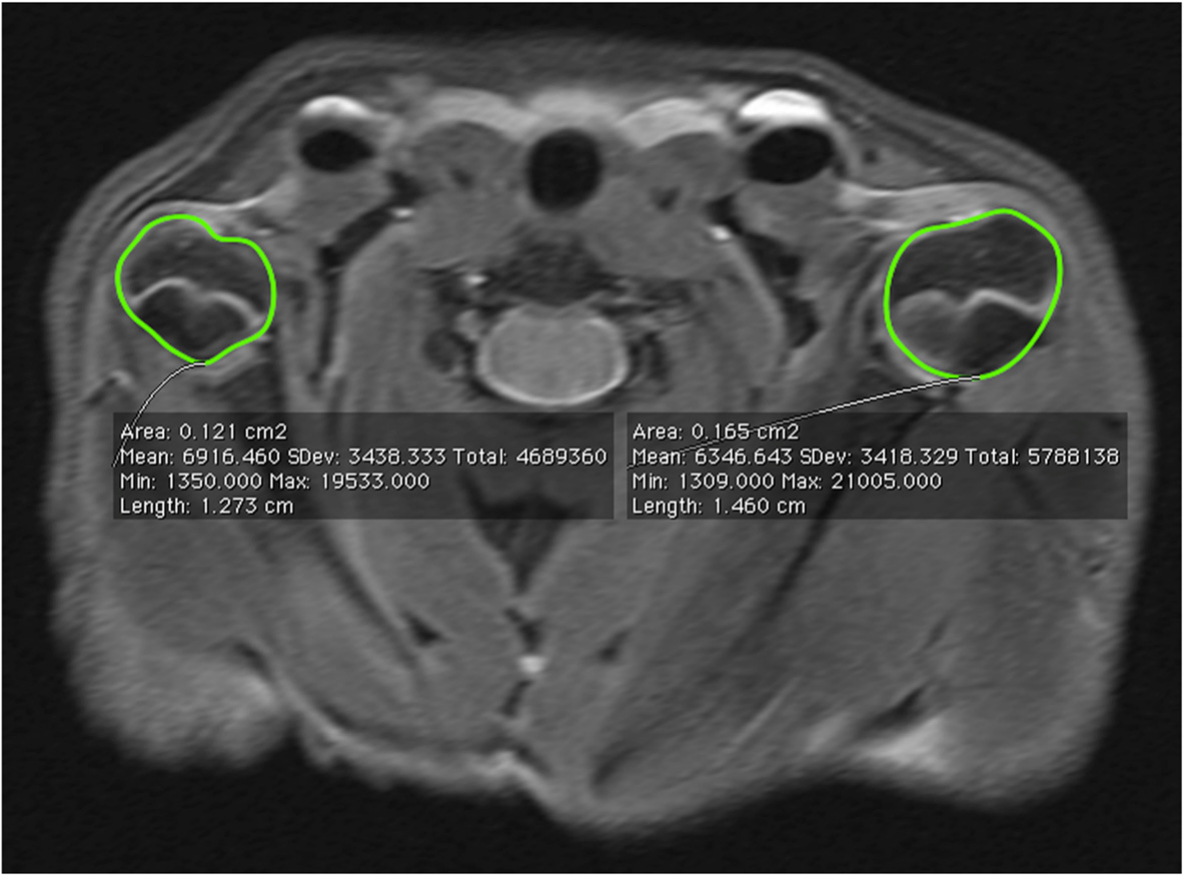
Shoulder MRI 8 weeks after surgery showing a decrease of humeral head sectional area that reproduces the joint hypoplasia found in children.
